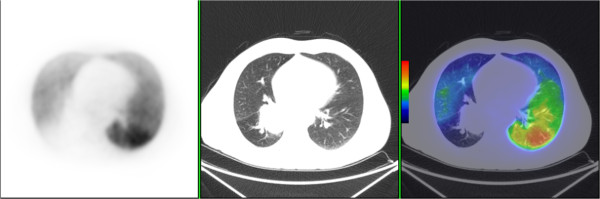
MAA-perfusion PET (left), contemporaneous CT (middle), and co-registered perfusion PET/CT, in a patient with a right upper lobe T3 squamous cell carcinoma.
